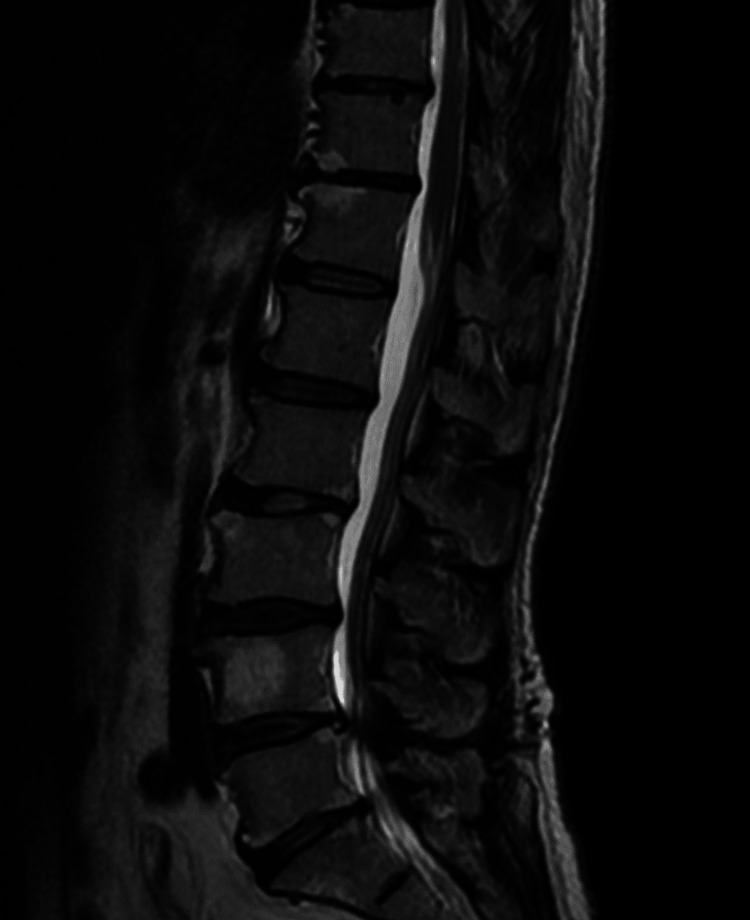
The result of lumbar magnetic resonance imaging (MRI). Lumbar MRI showing a herniation at the L4/5 level.
